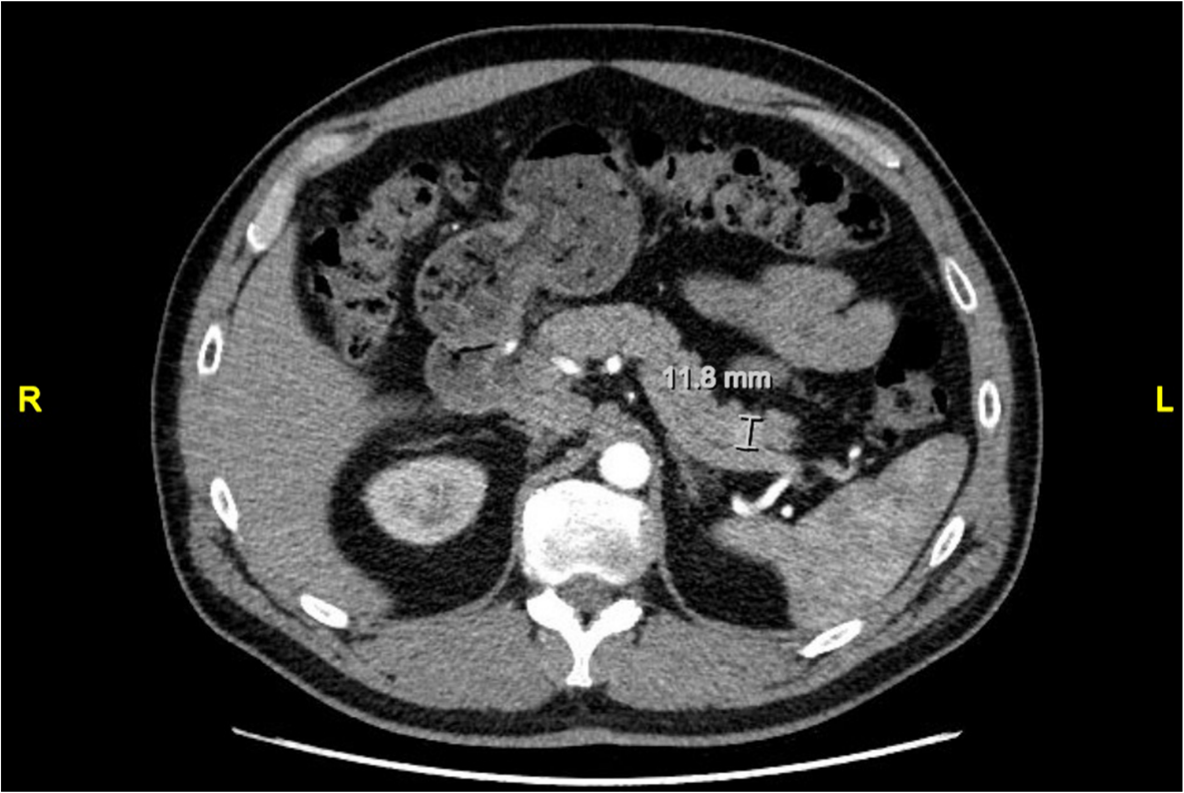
Computed tomographic scan of the abdomen showing a 1.2-cm tumor in the tail of the pancreas
